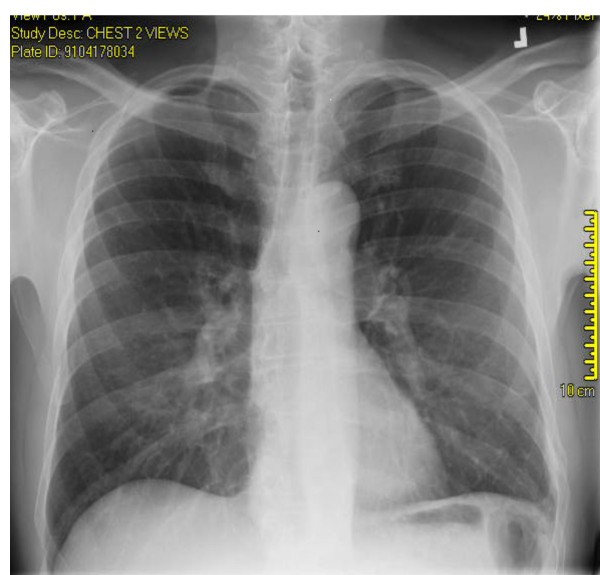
Chest X-ray at follow up: 5 months after repair of diaphragmatic hernia.
